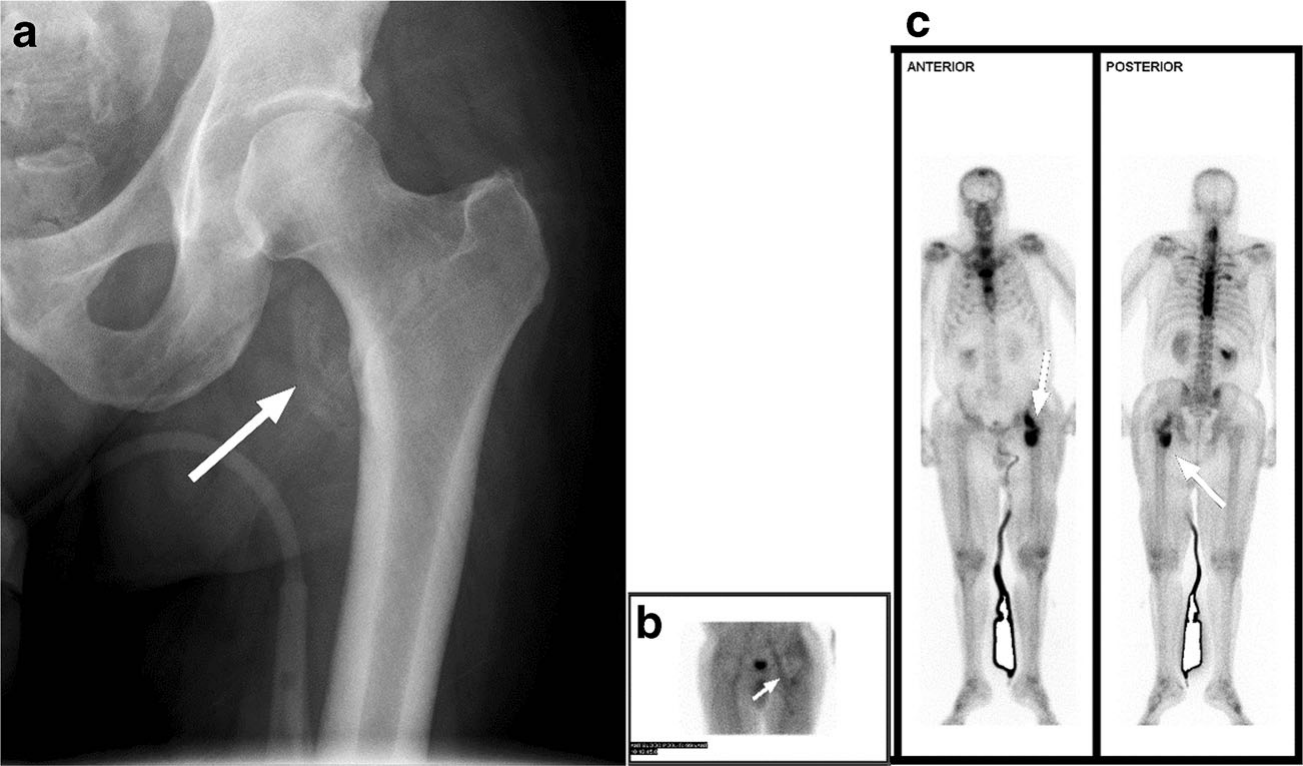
Heterotopic ossification. a Hip radiograph of a 46-year-old male 3 months after T4 SCI shows ill-defined calcifications medial to the lesser trochanter (*white arrow*). b Blood pool image of the subsequently obtained triple-phase bone scintigraphy shows hyperaemia in the left groin (*white arrow*). c Delayed bone scintigraphy images confirm avid tracer uptake medial to the left proximal femur (*white arrows*). Bone scan findings confirm maturing heterotopic ossification
